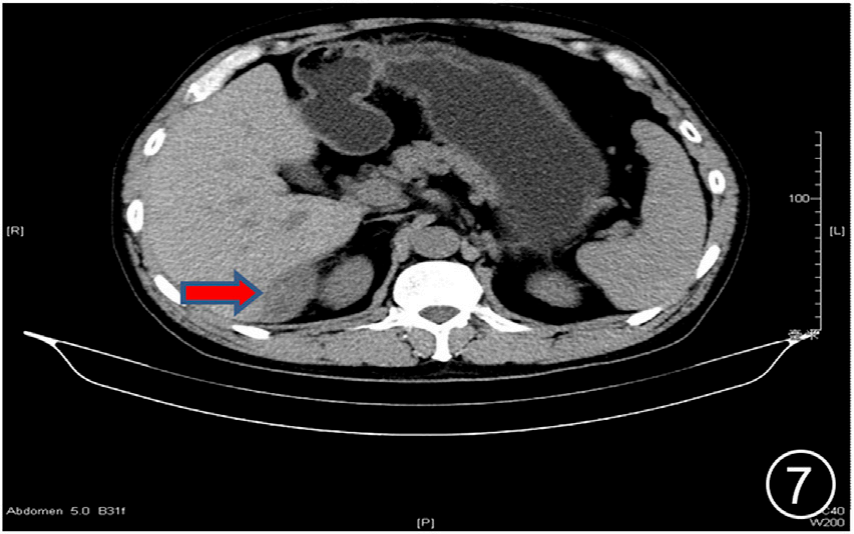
After six rounds of chemotherapy and four rounds of immunotherapy, the repeat CT showed uneven liver surface and the lesion in the liver-kidney space was significantly smaller than before.
